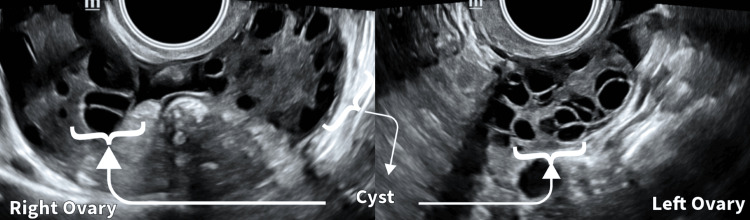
Pre-intervention transvaginal sonography image of the patient's right and left ovaries on day 2 of menstrual cycle. Multiple cysts in the right and left ovaries are depicted.
